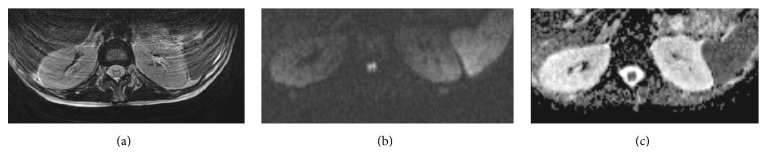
(a) The sagittal T2-weighted image shows a hyperintense lesion of the conus medullaris. (b) High signal is observed at the corresponding level on axial DWI. (c) Low ADC value on the ADC map confirmed the hypothesis of spinal cord infarction.
